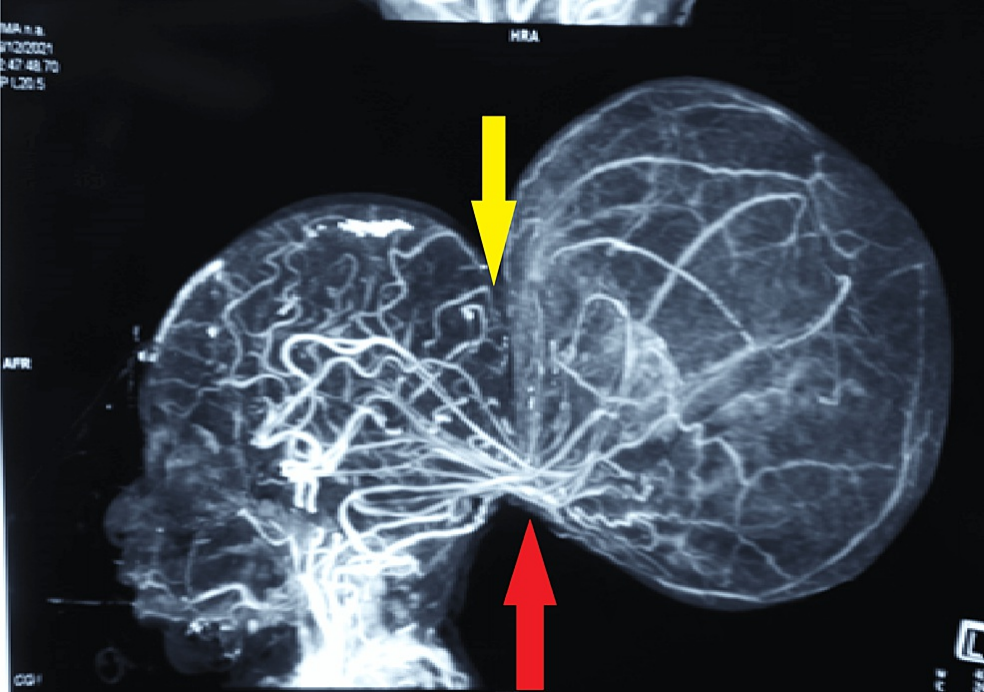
CT angiogram of the brain vessels. Yellow arrow showing a defect in the occipital bone. Red arrow showing intracranial vessels entering into the sac and looping out of the sac. CT - Computed tomography.
